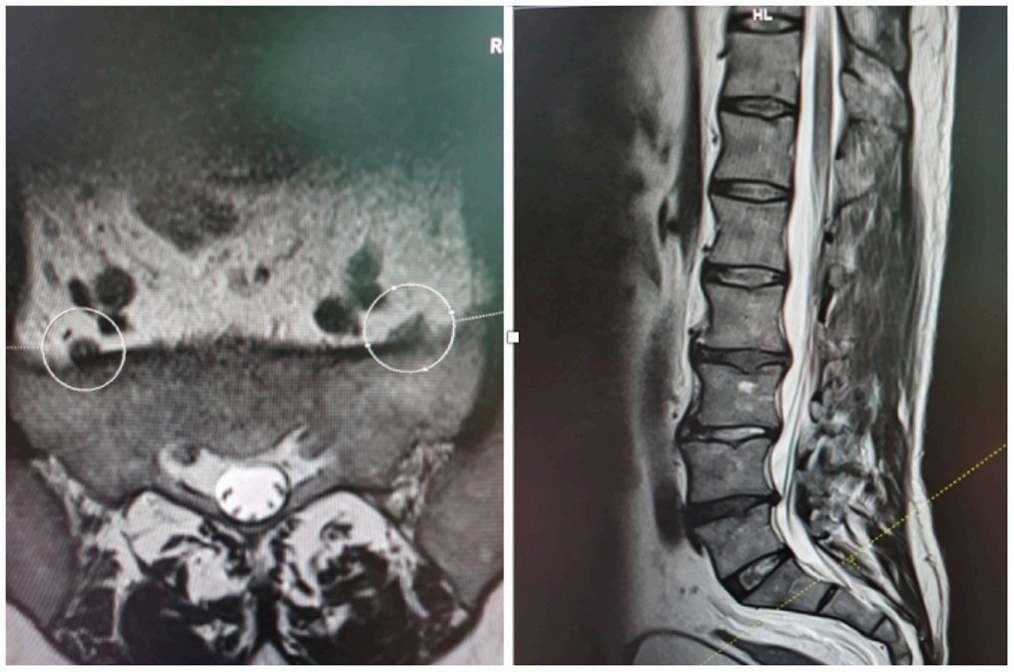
MRI showing transverse and sagittal projections with level of point B of SIJ.
